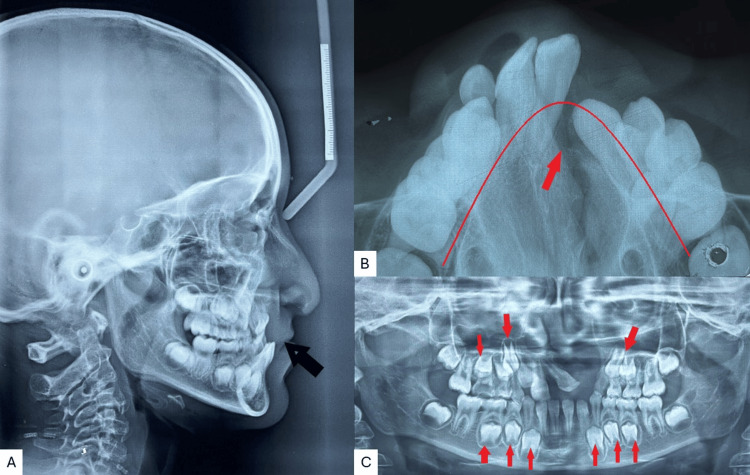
Pre-treatment radiographs A) Lateral cephalogram; B) maxillary occlusal; C) orthopantomogram (OPG)
